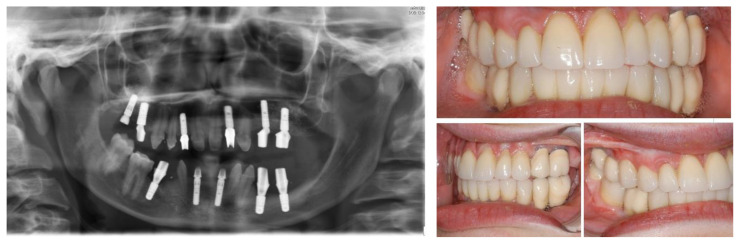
Left: final orthopantomogram showing the dental implants. Right: intra-oral situation after final rehabilitation with dental-supported and implant-supported prosthesis [12].
